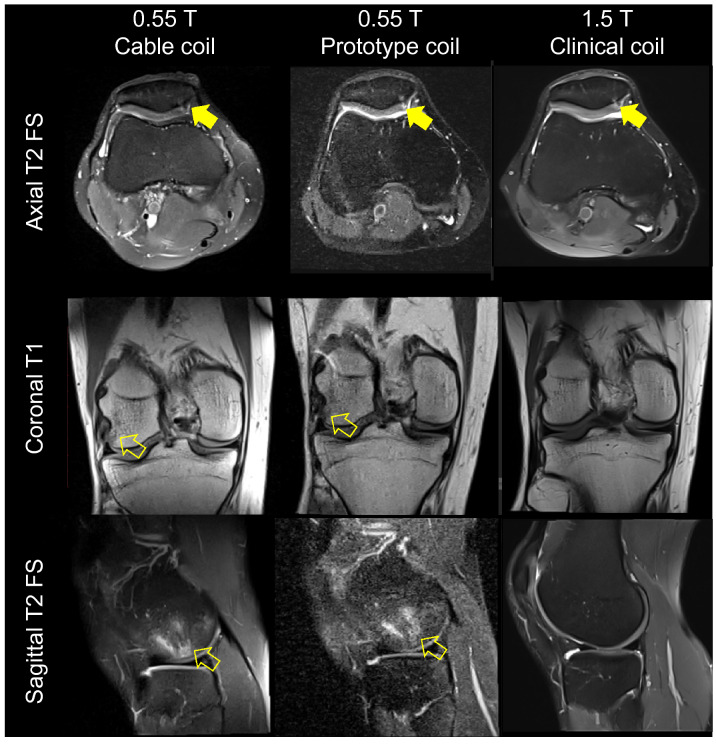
Turbo spin echo MR images of a 34-year-old man acquired using the 0.55 T cable coil (left column), 0.55 T prototype coil (middle column) and 1.5 T clinical coil (right column). The images reveal patellar cartilage defects (solid arrows) and a lateral femoral condyle bone contusion (open arrows). The bone contusion was not present at the time of the 1.5 T examination.
